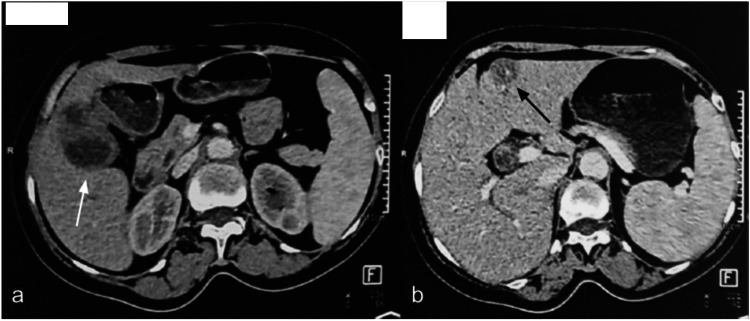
First abdominal contrast-enhanced computed tomography scan showing two liver abscesses. (a) Abscess lateral to the gallbladder (white arrow); (b) Abscess in the left lobe (black arrow). No underlying cause was identified (appendicitis, cholecystitis, tumor, foreign body).
